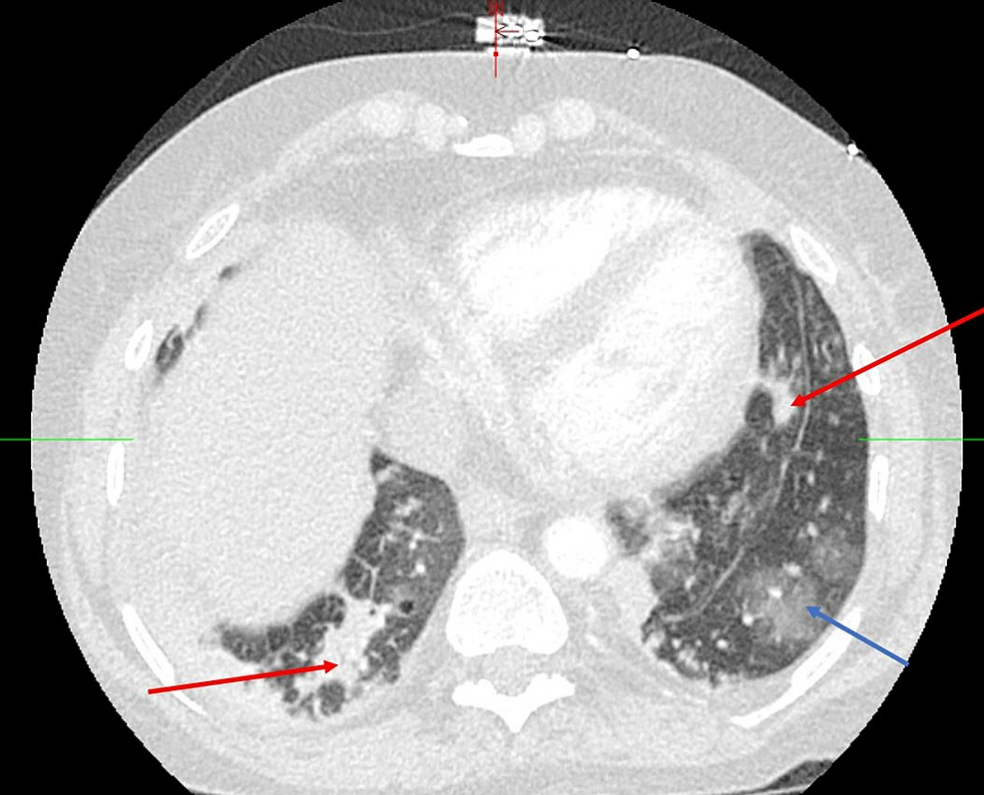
Computed tomography angiography chest on hospital day 9.
